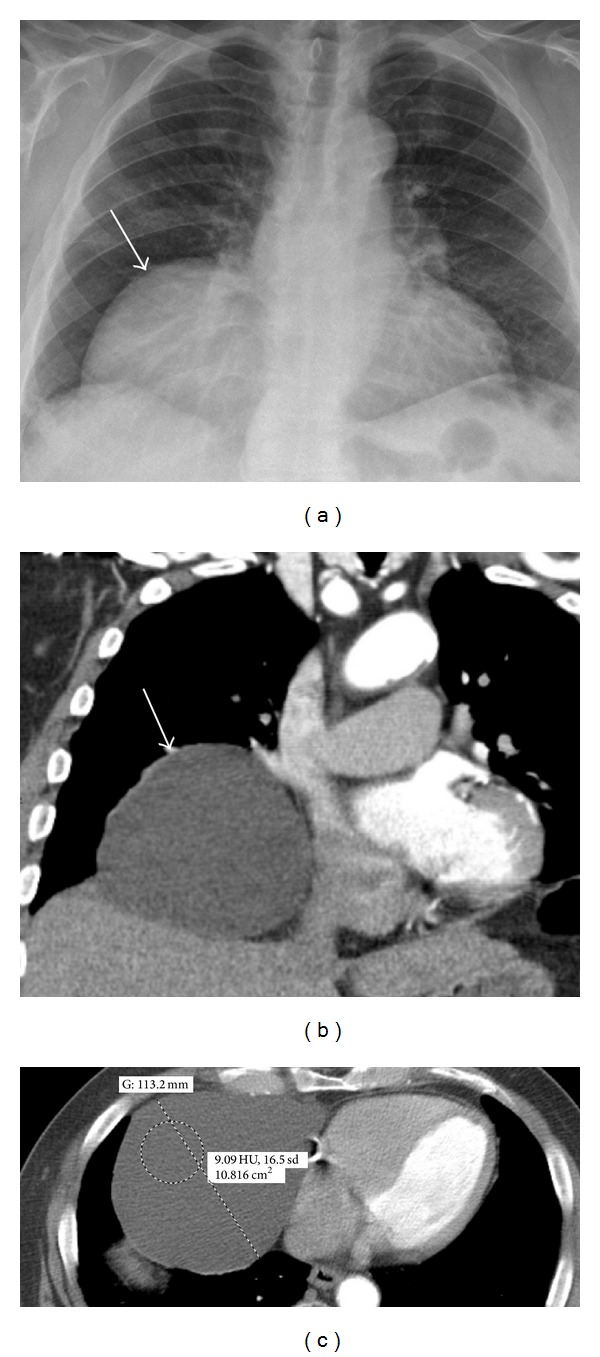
A 77-year-old male with shortness of breath. Chest radiograph (a) demonstrates an abnormal cardiac silhouette with prominence of the right cardiac border (arrow). The subsequent CECT (b, c) demonstrates an 11 cm pericardial cyst (arrow) containing simple fluid (HU of 9) without mass effect upon the SVC or IVC. Dyspnea was more likely related to early emphysematous and interstitial pulmonary changes demonstrated on the CT (not shown here).
